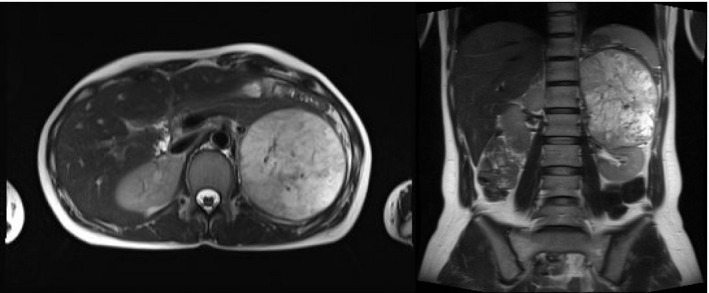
Abdominal magnetic resonance imaging revealing a large 11 × 8.5 cm left adrenal tumor.
